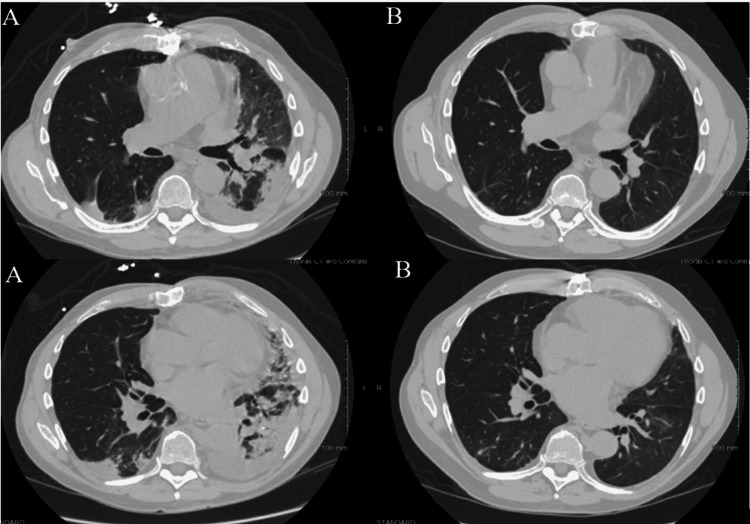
Chest CT upon presentation (A) shows left worse than right consolidative and ground-glass infiltrates and upon follow-up at six weeks (B) shows near-complete resolution of the infiltrates. CT: computed tomography.
